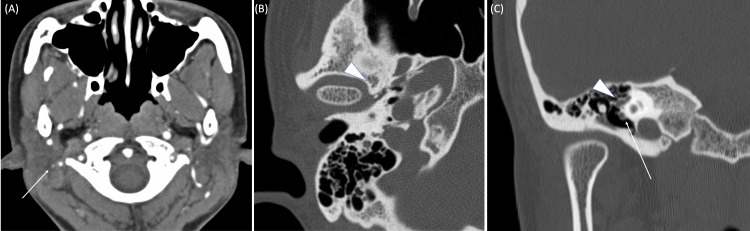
Axial and coronal contrast-enhanced CT scan of the right temporal bone. (A) The white arrow indicates a blood vessel from the branch of the right external carotid artery. (B) The white arrowhead indicates a defecting of the spinous foramen. (C) The white arrowhead indicates a vascular structure of the promontory. The white arrow indicates an enlargement from the facial nerve tympanic segment to the labyrinthine segment.
